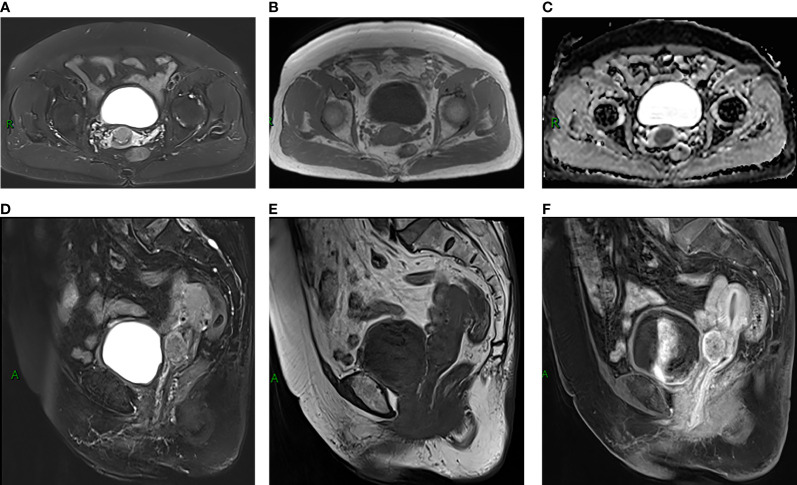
(A, B, D, E) MRI scans showed a well-defined oval mass located in cervix, which destroyed the stroma (A). The mass showed slight hyperintensity on T2-weighted MR images (A, D) and isointensity on T1-weighted MR images (B, E). (C) The ADC map showed low ADCs. (F) Sagittal contrast-enhanced MR image showed mild to moderate heterogeneous enhancement of the lesion, presenting rim-enhancement.
